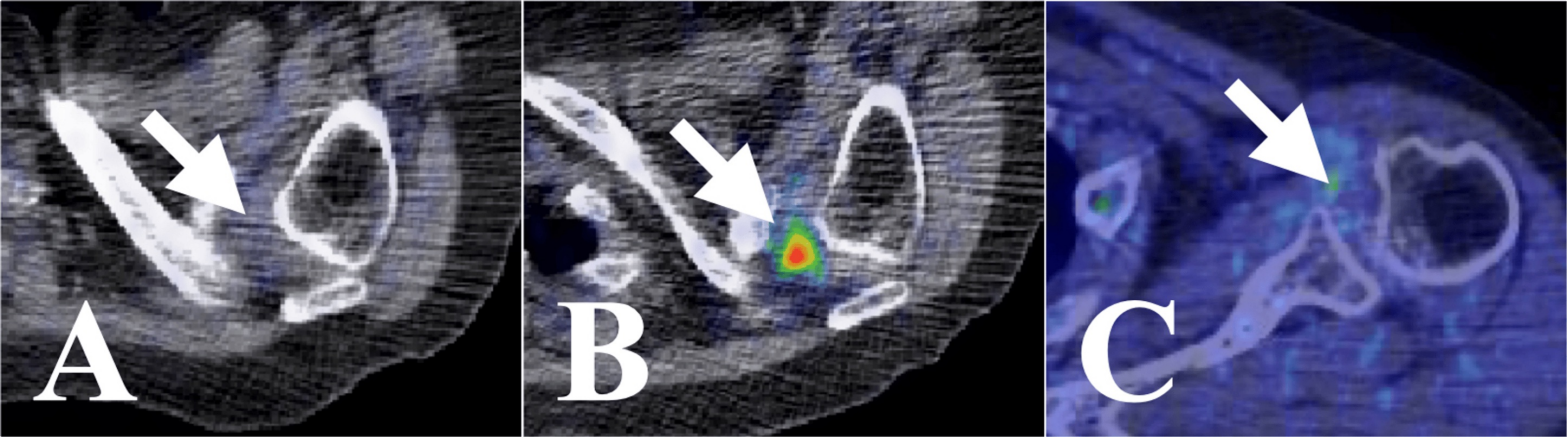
Case 2. Axial FDG-PET/CT findings. (A) Two years and five months before CK-SBRT, a PET/CT scan showing no FDG uptake in the left shoulder joint. (B) Two months before CK-SBRT, a PET/CT scan showing high focal FDG uptake at the rotator interval of the left shoulder joint. (C) Thirteen months after CK-SBRT, a PET/CT scan showing no FDG uptake in the left shoulder joint. FDG: 2-deoxy-2-[18F] fluoro-D-glucose; PET: positron emission tomography; CT: computed tomography; CK-SBRT: stereotactic body radiotherapy using CyberKnife
